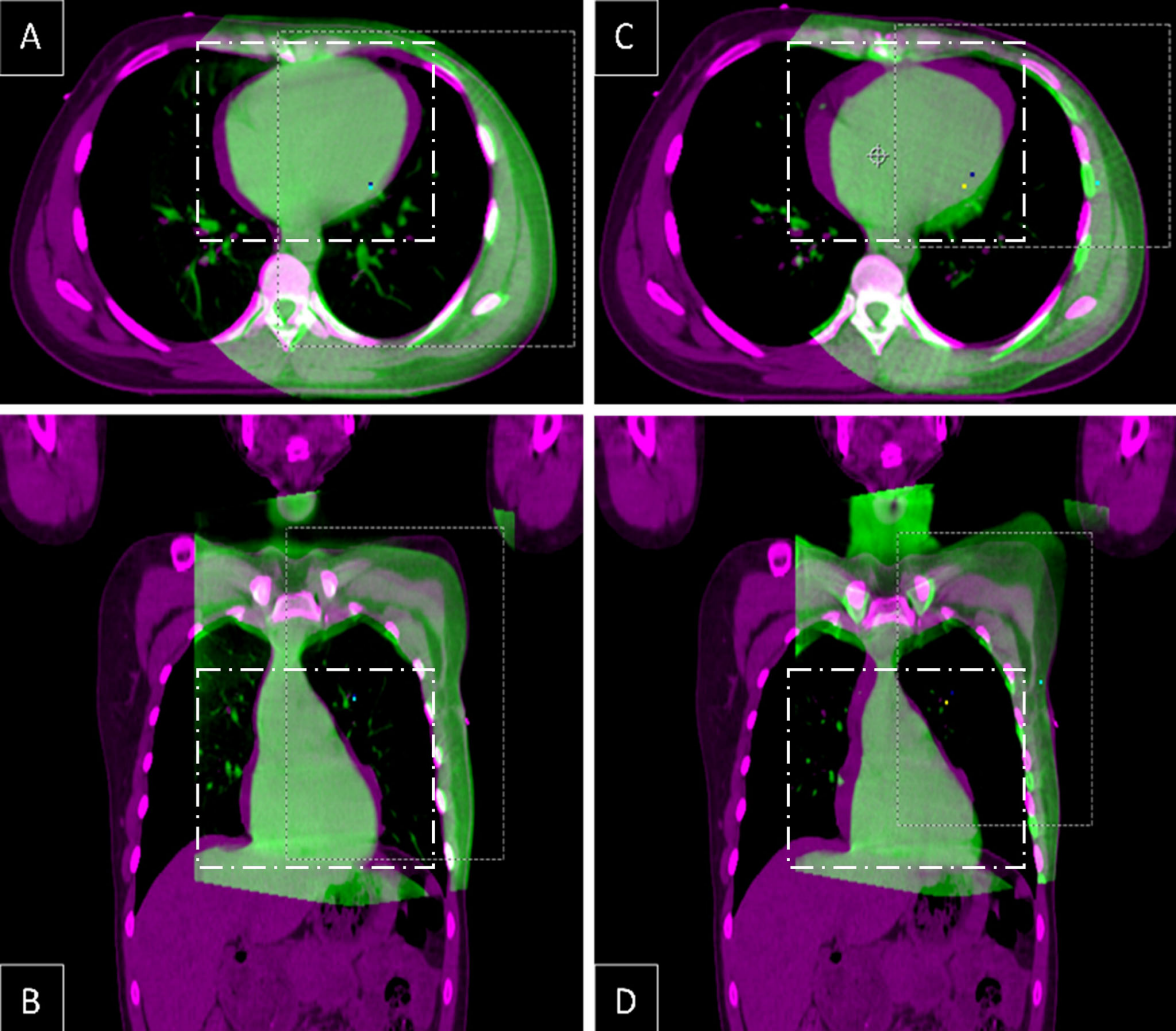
Fusion of axial (A, C) and coronal (B, D) CT slices from the same level in DIBH planning CT (pink) and the first (A, B) and the last (C, D) CBCT (green), showing interfractional heart translation.
